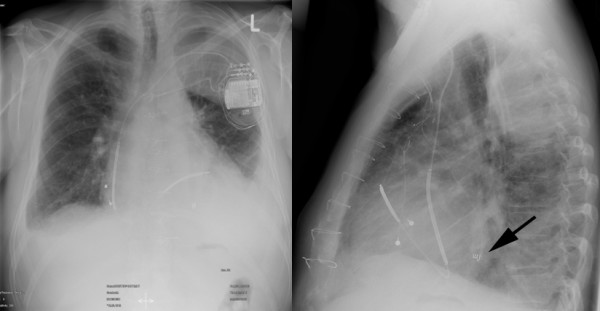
Postoperative x-ray demonstrating location of the transvenous ICD electrode, the left ventricular epicardial screw-in electrode at the lateral wall (arrow) and the bipolar epicardial lead at the right atrium.
